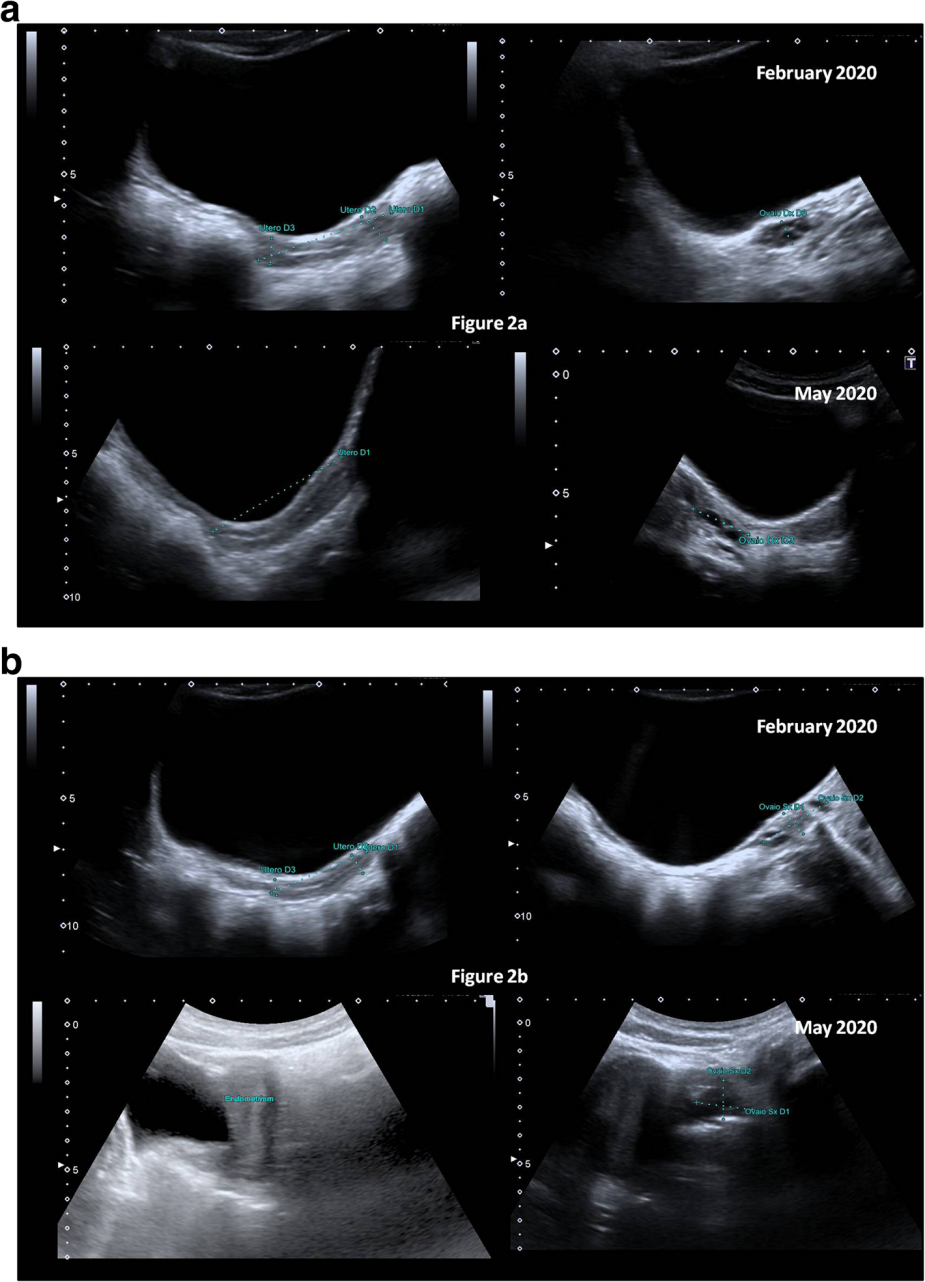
Two girls with central precocious puberty with a slow rate progression before lockdown and acceleration during or after lockdown for COVID-19. Patient 1 started puberty at 7.5 years (November 2019: height 1.23 SDS, BMI 0.88 SDS; Tanner stage B2 PH1 AH1); the second pelvic sonography, February 2020 (a) is similar to the sonography at diagnosis (uterine length 43 mm and ovary volumes respectively 1.8 and 1.2 mL); the sonography in May 2020 (b) showing an acceleration in the tempo of puberty (height 1.29 SDS, BMI 0.91 SDS; Tanner stage B3–4 PH2 AH1; uterine length 52 mm, ovaries volume 4,9 and 4,0 mL). Patient 2 started puberty at 7.4 years (October 2019: height 1.17 SDS, BMI 0.69 SDS; Tanner stage B2 PH1 AH1); the second pelvic sonography in February 2020 (b) is similar to the sonography at diagnosis (uterine length 45 mm and ovaries volume respectively 3.8 and 3.7 mL); the sonography in May 2020 shows also an acceleration in the tempo of puberty (height 1.22 SDS, BMI 0.76 SDS; Tanner stage B3–4 PH1 AH1; uterine length 63 mm and ovary volumes respectively 6.4 and 8.8 mL)
